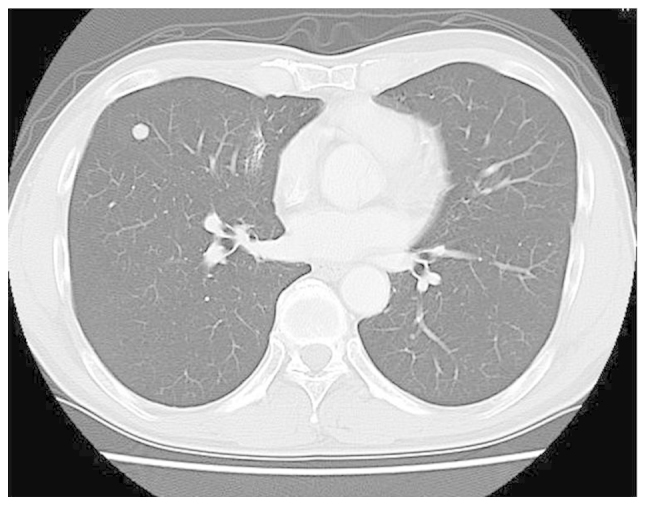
Lung computed tomography showing small nodules of lung metastases.
